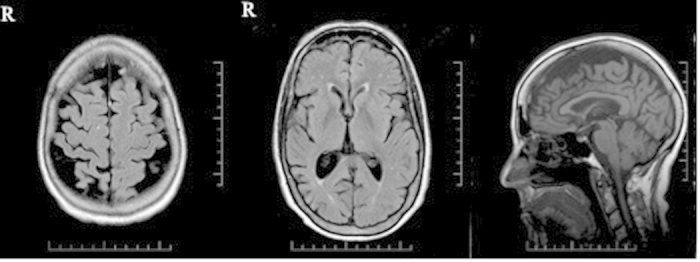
T1-weighted MRI of the brain of patient 10162.
